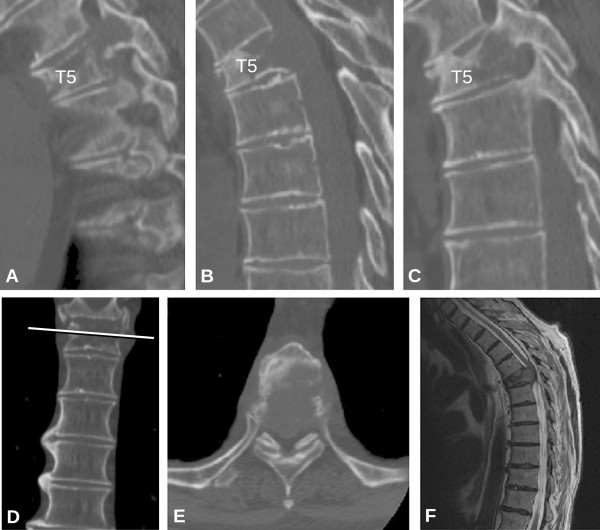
A 67-year-old man with known metastatic small cell lung cancer presents with interscapular back pain that is exacerbated by movement and relieved with rest. Computed Tomography (CT) (A: left parasagittal, B: midline parasagittal, C: right parasagittal, D: coronal, and E: axial) and Magnectic Resonance (MR) images (F: sagittal, T2-weighted) outline the key details of this T5 lesion. Total SINS score = Semirigid (T5) spine, 1 point; ‘Mechanical’ pain (yes), 3 points; Lytic lesion, 2 points; De novo kyphotic deformity without subluxation/translation, 2 points; >50% vertebral body collapse, 3 points; and bilateral posterolateral spinal element involvement, 3 points. Total SINS score = 1 + 3 + 2 + 2 + 3 + 3 = 14 (unstable, surgical consultation is recommended).
